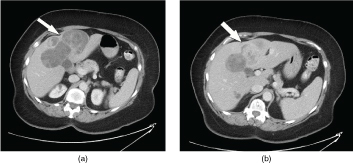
(a,b) Multiple axial CT images with contrast through the liver demonstrate a heterogeneous, predominately cystic, partially subcapsular mass (white arrows) centred in the anterior segment of the right hepatic lobe.
